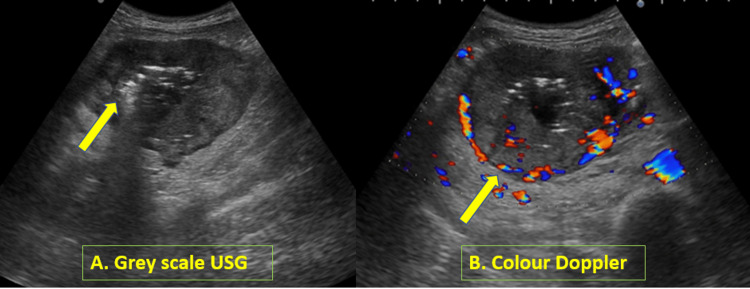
(A) B-mode grayscale ultrasound (USG) image depicting a hypoechoic lesion with central hyperechoic foci (shown by the arrow) representing air bubbles. (B) A color Doppler image showing vascularity in the lesion, more toward the periphery (shown by the arrow), with central necrosis and air foci.
